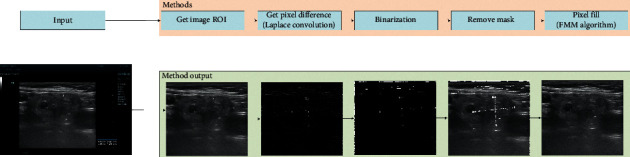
Ultrasonic image removal method and output.
